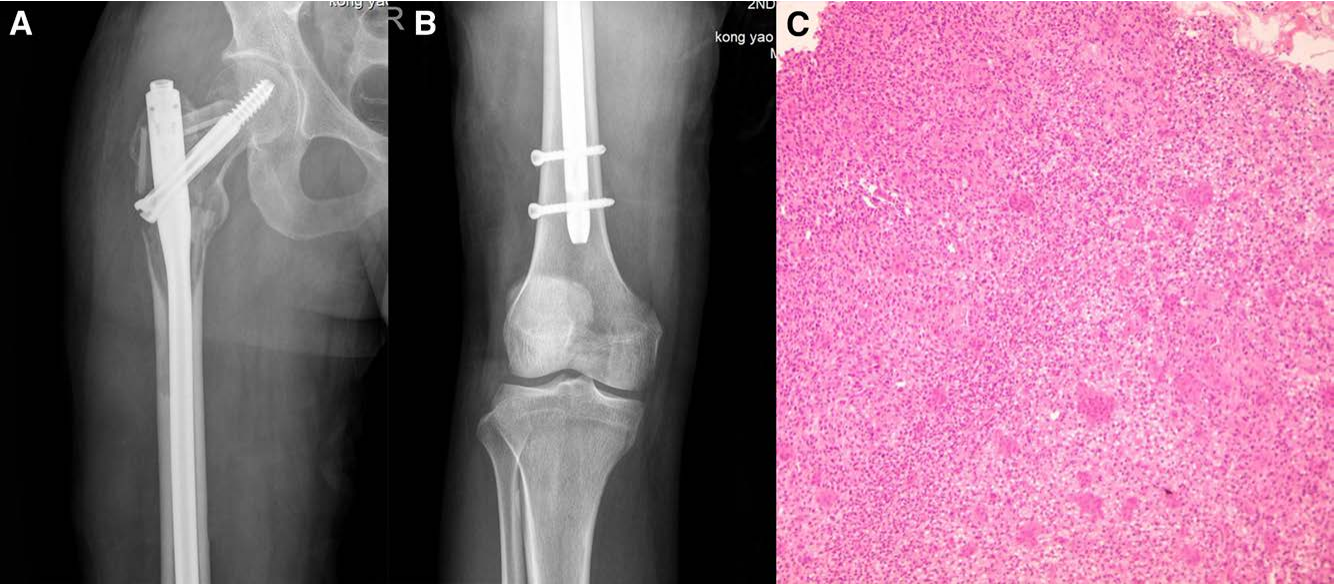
(A and B) Postoperative anteroposterior radiographs showed that the allograft fibula were in good position at 3 sites after intramedullary nail-fixation. (C) A tumor rich in giant cells (HE × 100).
